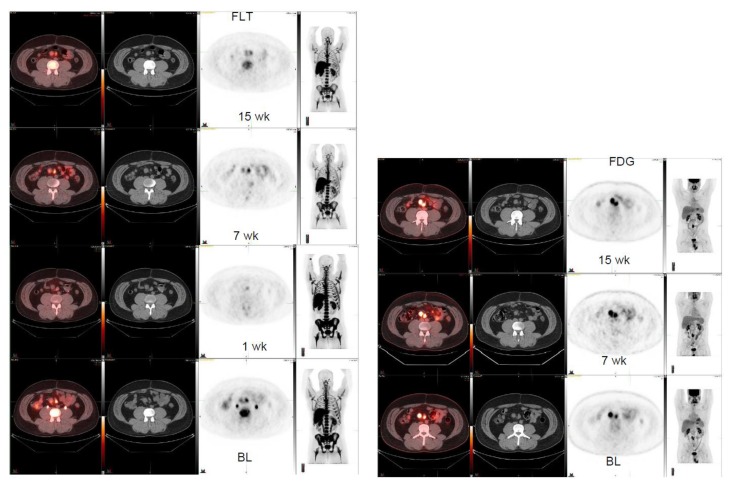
Gastro neuroectodermal tumor (GNET). FLT-study at baseline, at 1, at 7, and at 15 weeks (left panel, 4 rows). In the right mesenteric lymph node, FLT varies: SUVmax 5.2 ->3.2 -> 3.9->2.8 whereas corresponding sizes change on CT as follows: 2.2 cm × 1.9 cm ->2.0 cm × 1.8 cm->2.0 cm × 2.0 cm ->1.9 cm × 1.7 cm. In the left mesenteric lnn, FLT varies: SUVmax 5.5 ->3.4 -> 5.9->4.0 whereas corresponding sizes change on CT as follows: 2.3 cm × 1.6 cm ->1.9 cm × 1.5 cm->2.1 cm × 1.4cm ->2.0 cm × 1.2 cm. FDG-study at baseline, at 7, and at 15 weeks (right panel, 3 rows). In the right mesenteric lnn, FDG varies: SUVmax 12.9 ->nm -> 15.4->10.6 and in the left mesenteric lnn: SUVmax 8.2 ->nm -> 9.9->8.0.
